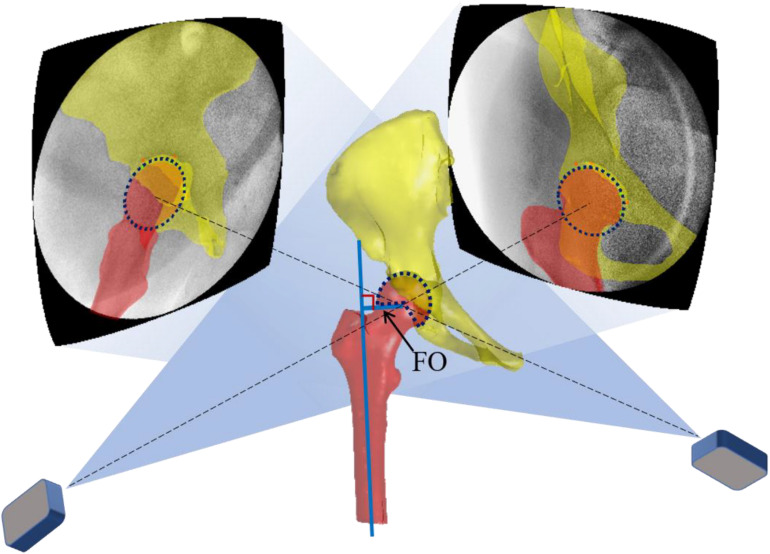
A virtual DFIS environment is constructed following the projection parameters obtained from the calibration procedure. The two-projection line intersection from two X-ray sources to the hip joint center determines the 3D skeletal models’ position. The FO was defined as the perpendicular distance from the femoral head’s rotation center to the femoral long axis.
